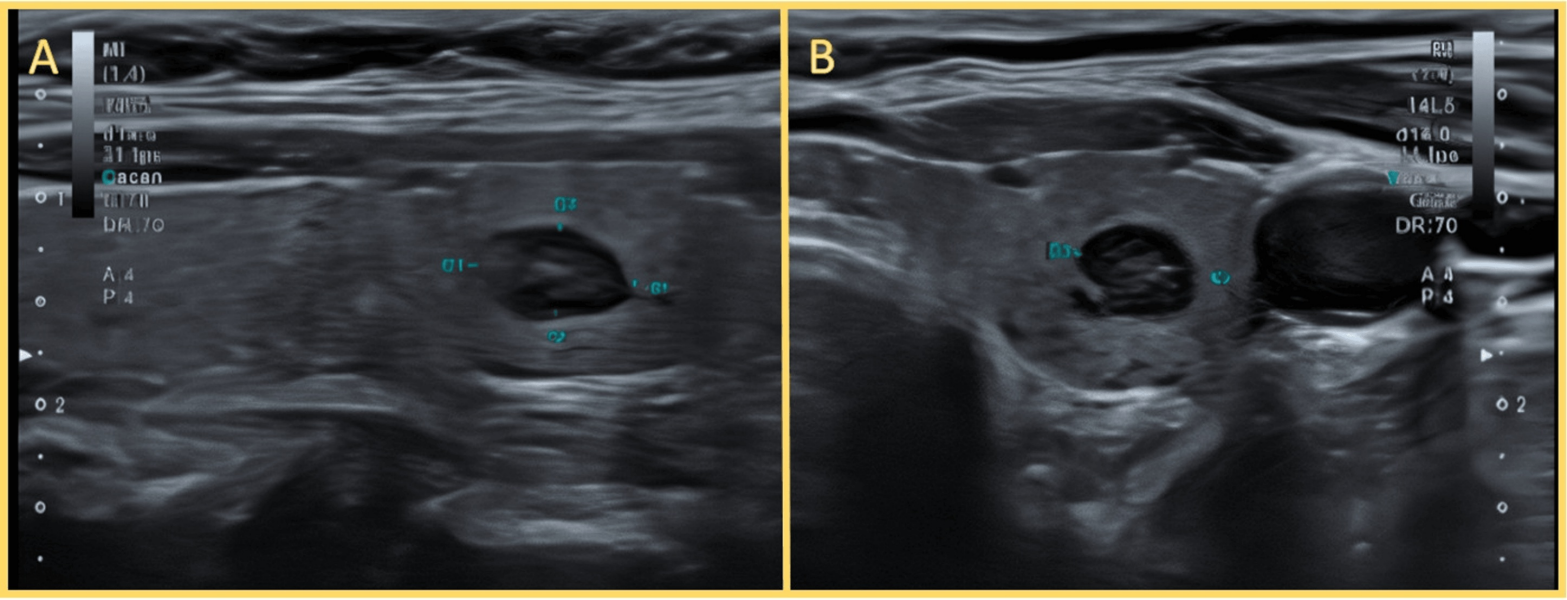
Thyroid ultrasound showing suspicious nodule in the left lobe (A, B) Transverse views of the left thyroid lobe demonstrating a hypoechoic, solid nodule with irregular margins and taller-than-wide orientation. The lesion measures approximately 0.5 cm and was classified as TIRADS 5 due to its ultrasonographic features. Fine-needle aspiration yielded Bethesda category VI cytology, confirming papillary thyroid carcinoma.
